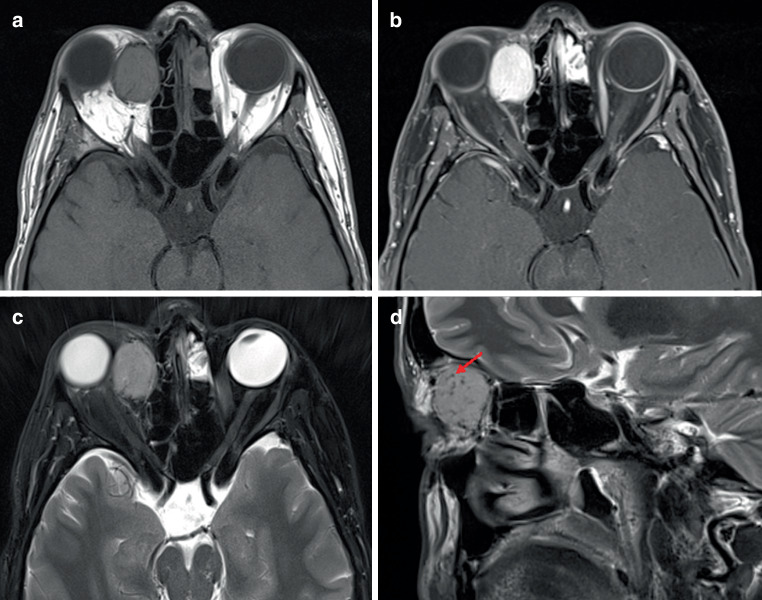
Magnetic resonance imaging of right-sided orbital solitary fibrous tumor (SFT) in a 29-year-old male. a axial plane of non-contrast T1-weighted imaging demonstrating similar signal intensity between SFT, brain cortex, and muscle temporalis, b axial plane of contrast-enhanced and fat-saturated T1-weighted imaging demonstrating markedly increased and homogeneous contrast uptake of the tumor, c axial plane of T2-weighted imaging with fat saturation showing similar signal intensity of the tumor and brain cortex, d sagittal plane of T2-weighted imaging demonstrating presence of tumor flow voids (*red arrow*) as a typical finding of SFT. *SFT* solitary fibrous tumor
